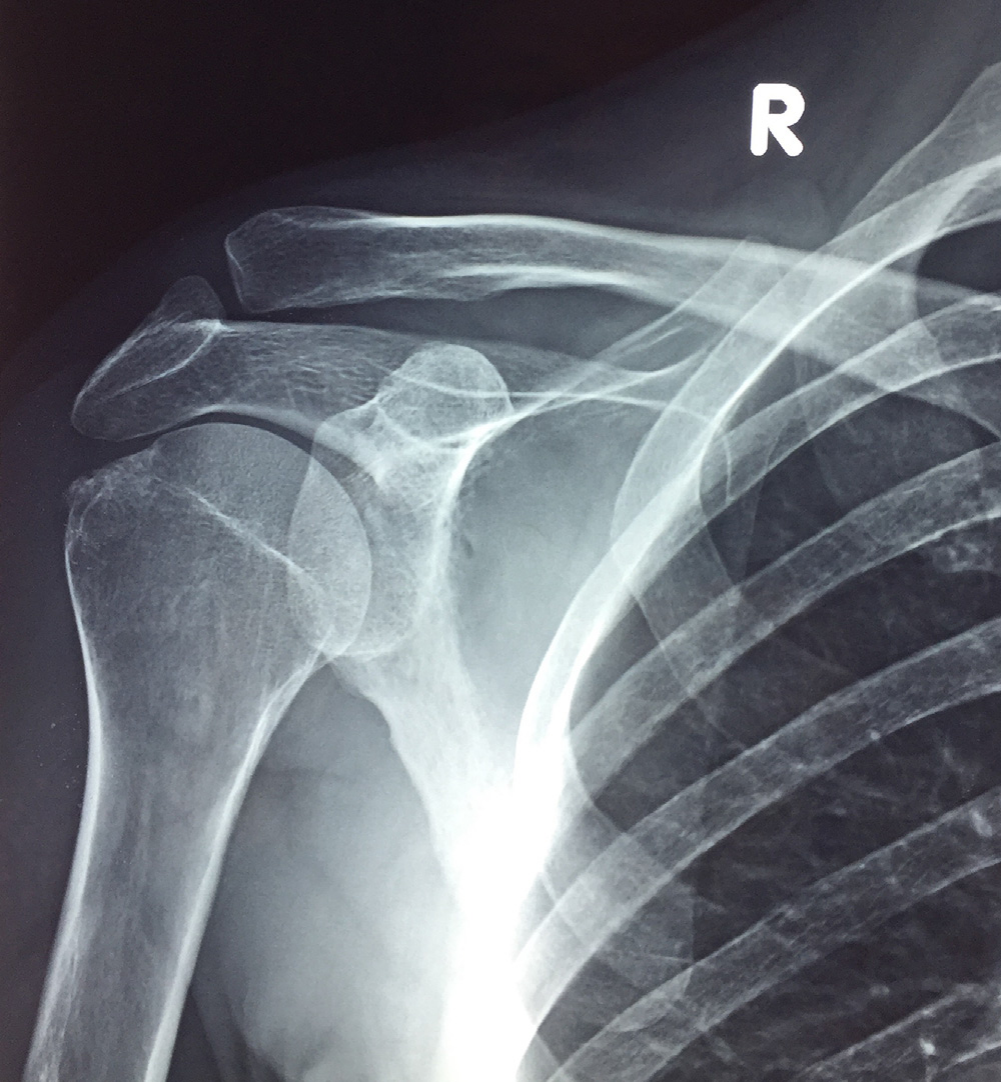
X-ray of the same patient 30 days after the needling and immediately after the end of the whole treating protocol.
